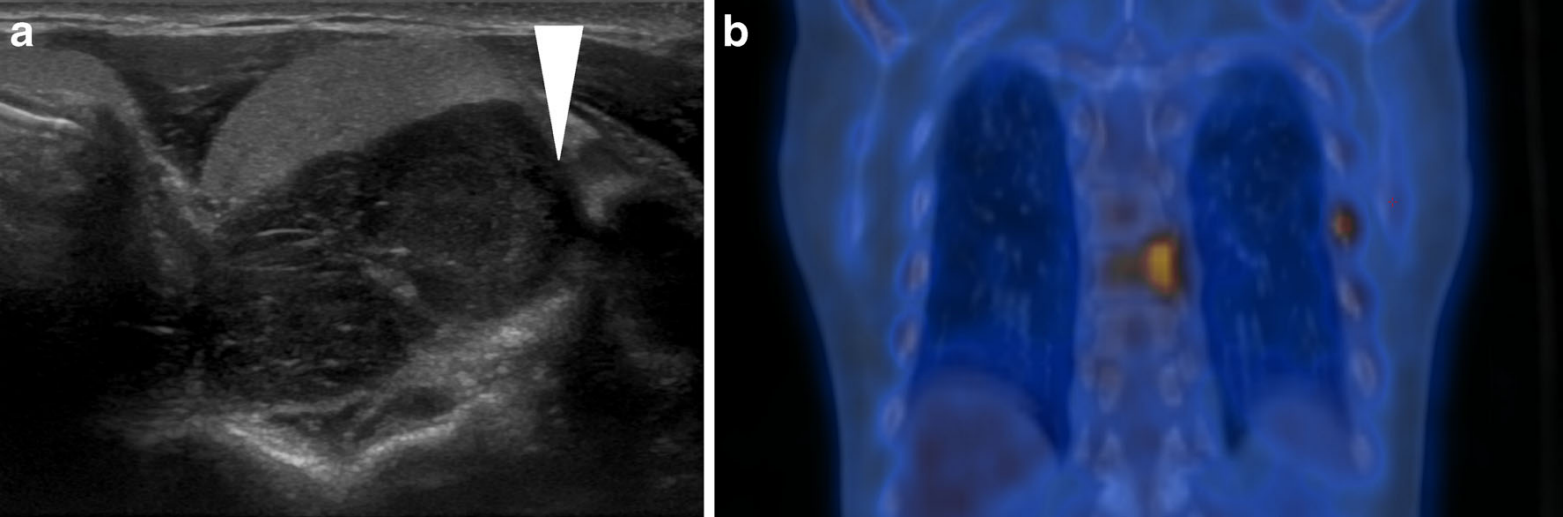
a) Transverse ultrasound image of thyroid and b) coronal FDG PET-CT of the thorax of a 50-year-old male with HPT-JT who has a parathyroid carcinoma and a bone metastasis at presentation. The ultrasound (a) demonstrates a hypoechoic parathyroid neoplasm (arrowhead) indenting the thyroid with findings suspicious for underlying invasion of the prevertebral musculature. The PET-CT demonstrates intense tracer uptake in the left posterior T8 vertebral body, which was proven on biopsy to be a metastatic deposit. Focal uptake in the left 7th rib is secondary to a rib fracture. HPT-JT patients have a greater predisposition to develop parathyroid carcinomas than the general populationiii.Renal and uterine tumoursWilms tumours, nephroblastomas, harmatomas, papillary renal cell carcinomas and an increased incidence of simple renal cysts have been reported in HPT-JT patients [15–17]. There is a reported higher incidence of uterine carcinomas and uterine polyposis in females with HPT-JT [17].
